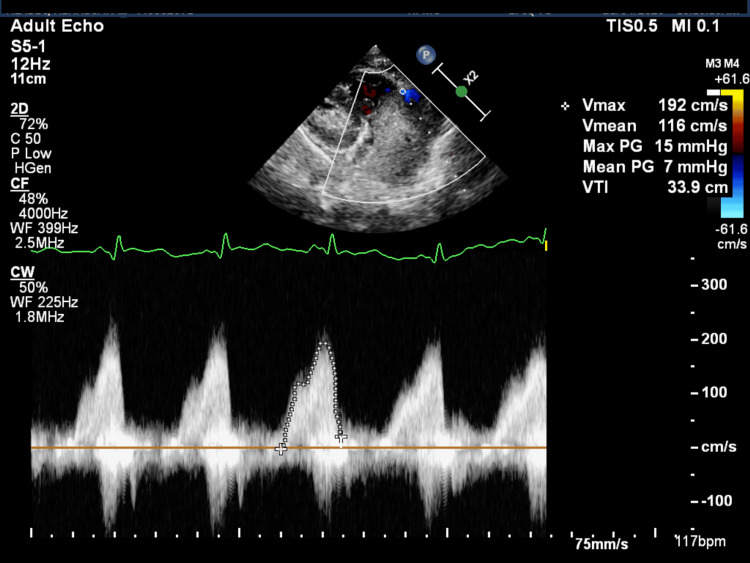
Parasternal long axis view of the heart with a continuous doppler The mean gradient across the tricuspid valve is seen in the echocardiogram above, showing a value of 7 mmHg, with a maximum of 15 mmHg, which is well above the normal expected gradient of <2 mmHg denoting severe obstruction.
